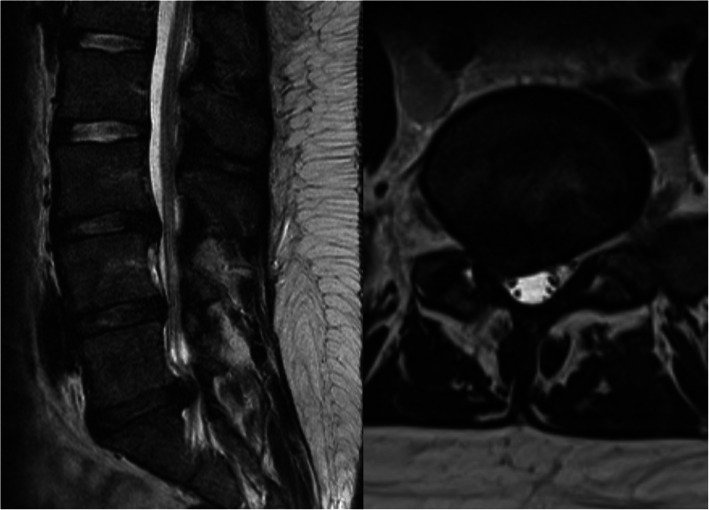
MRI showing lumbar disc herniation on L5-S1 disc with neural compression on right S1 nerve root (T2-weighted sagittal and axial views)
